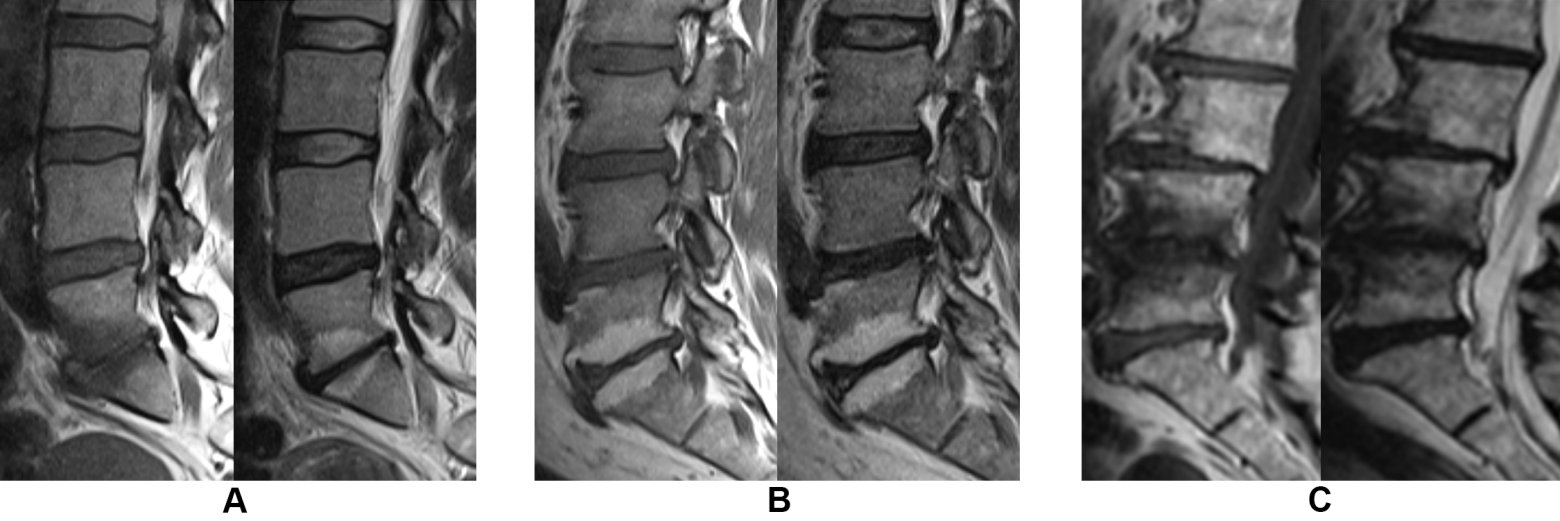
Modic changes on T1- (left) and T2-weighted (right) images from a 1.5 Tesla MRI scanner. (A) Modic changes type 1 at level L5-S1 (B) Modic changes type 2 at level L5-S1 (C) Modic changes type 3 at level L4-L5.
